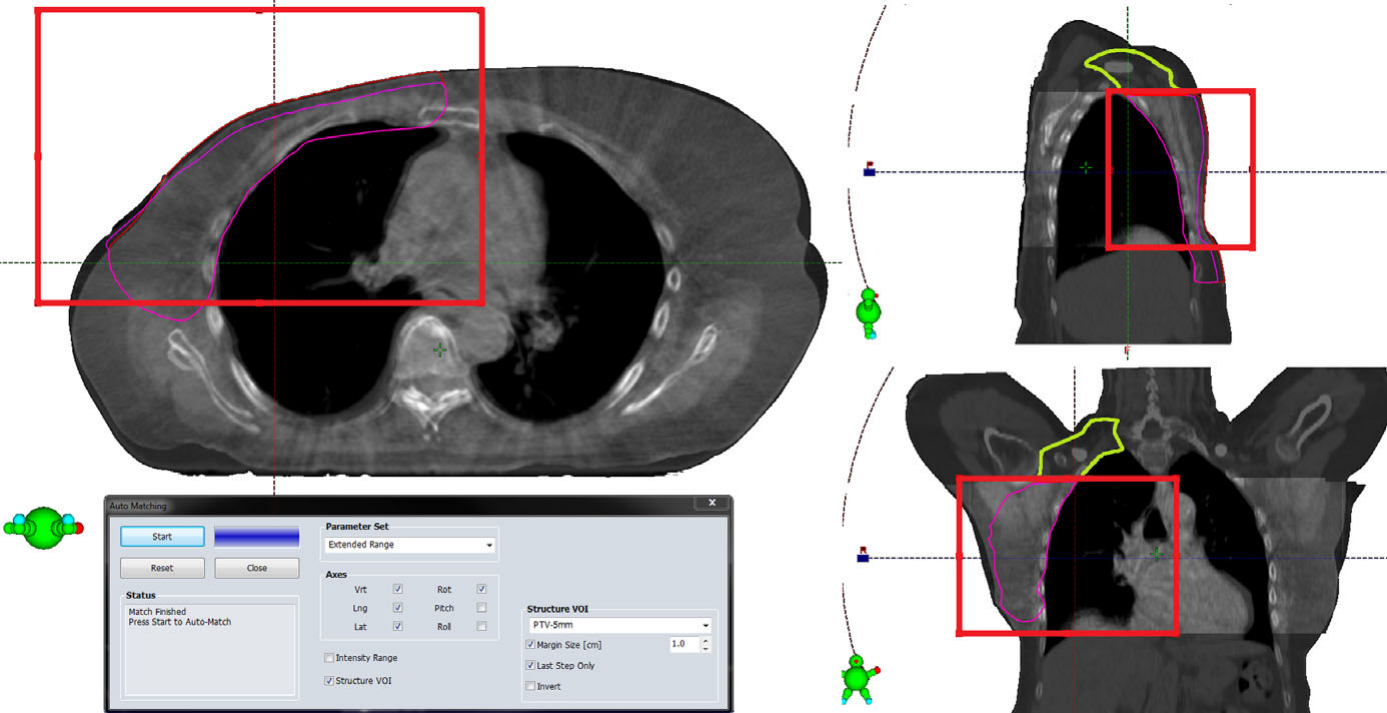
Region of interest for matching the CBCT image to the CT image is drawn with the red rectangle. The matching region of interest is centered to the PTV (red contour), effectively excluding the spine. The division of PTV‐5 mm into PTVb/c (magneta contour) and PTVsclav (green contour) is made on the level where the original PTV (red contour) reaches the skin, shown in the sagittal and coronal views.
